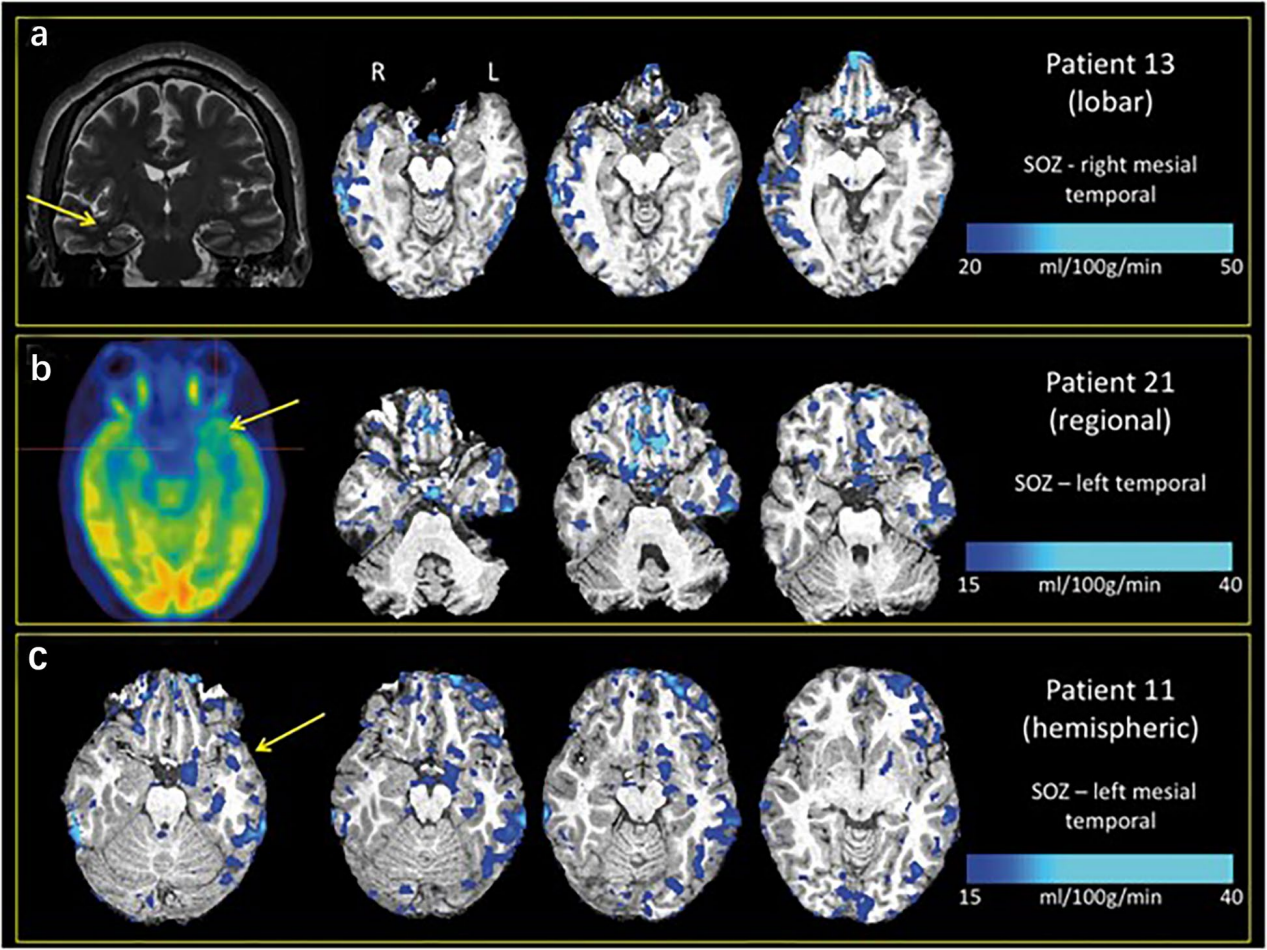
Examples of different patterns of postictal hypoperfusion seen on subtracted ASL. a Right mesial temporal sclerosis (arrow) on MRI and a "lobar" pattern of hypoperfusion on postictal ASL; the presumed seizure onset zone (SOZ) was in the right mesial temporal lobe. b Left temporal hypometabolism (arrow) on interictal PET image and a "regional" pattern of hypoperfusion on postictal ASL; the presumed SOZ was in the left temporal lobe. c No lesions on MRI and independent, bilateral mesial temporal SOZs based on intracranial VEEG monitoring, and a left "hemispheric" pattern of hypoperfusion on postictal ASL from a left mesial temporal seizure. Permission was granted by Gaxiola-Valdez et al. (© Oxford University Press [22]) to reuse this figure
